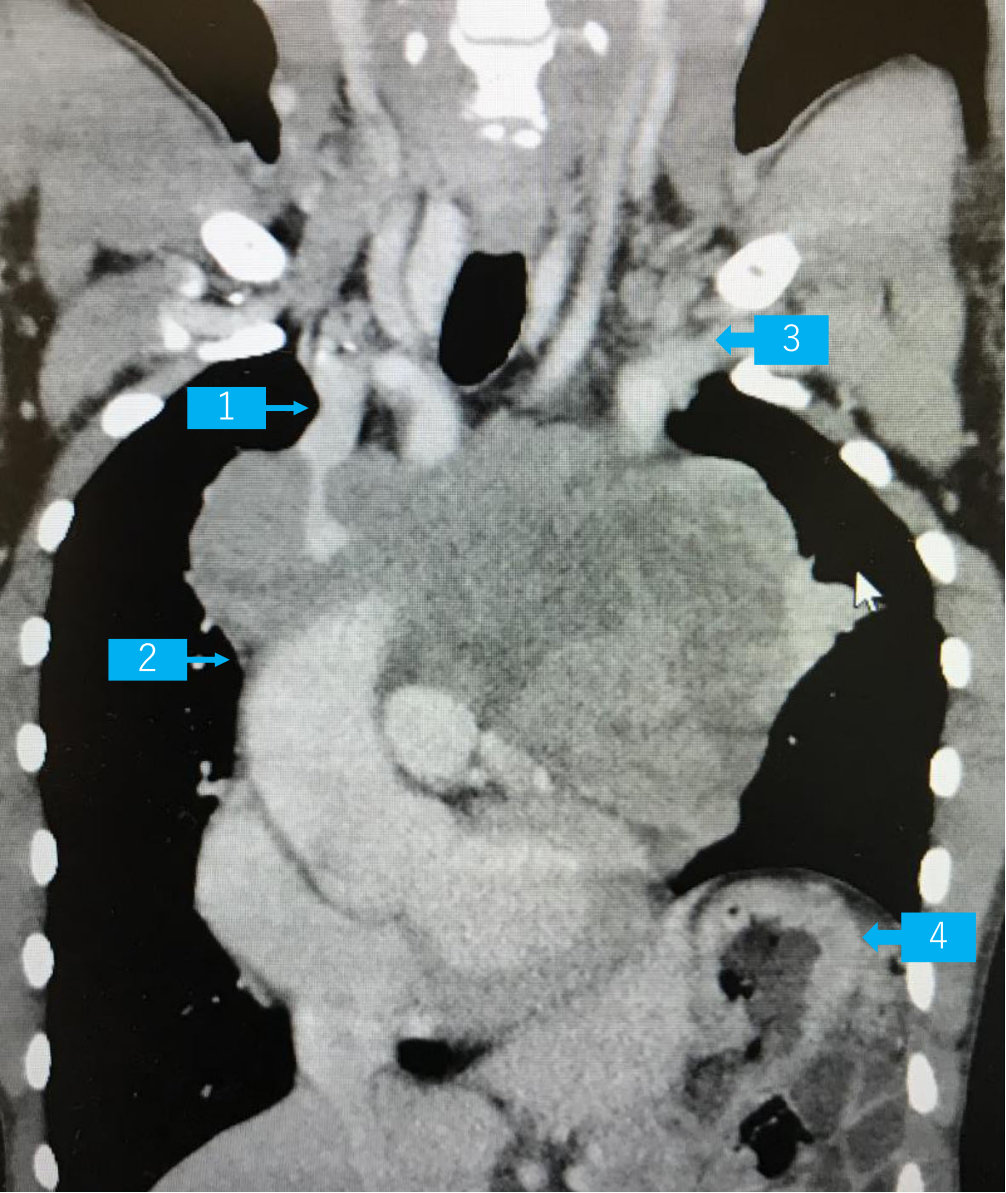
CT scan showing the location of the giant anterior mediastinal tumor. Arrow 1, right innominate vein; arrow 2, superior vena cava (SVC); arrow 3, left innominate vein; arrow 4, elevated left diaphragm
